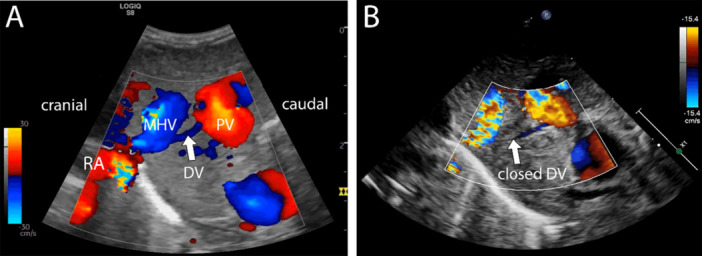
Color Doppler echocardiography (longitudinal view of the upper abdomen): (A) Patent DV showing severe constriction at the referring hospital (MHV = middle hepatic vein, PV = portal vein, RA = right atrium); (B) closed DV after transfer. [Color figure can be viewed at wileyonlinelibrary.com]
